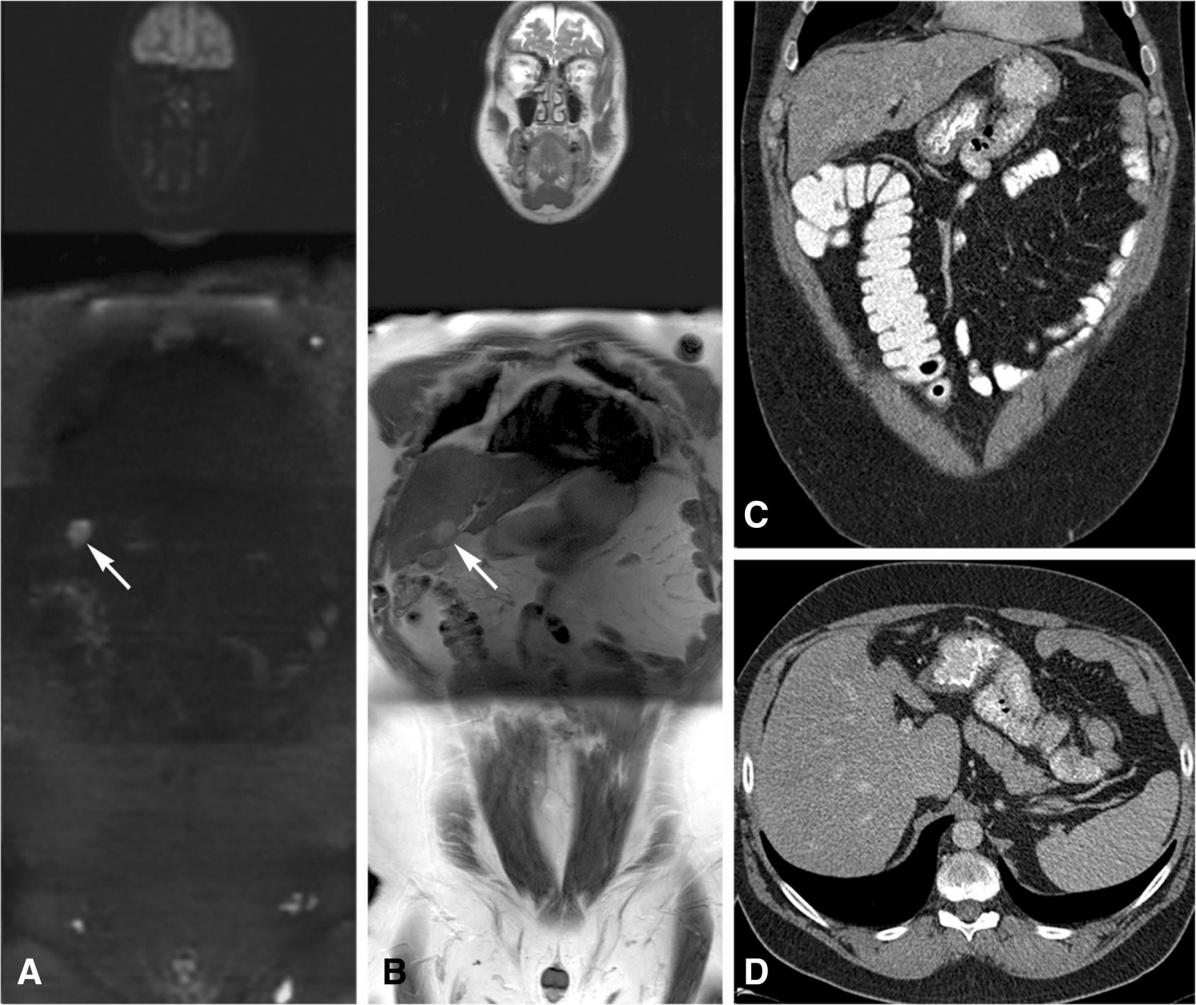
A 49 year old male patient with a history of a sigmoid resection because of adenocarcinoma was diagnosed with tumour recurrence. He had a WB-DWI/MRI for operability assessment. Apart from limited peritoneal disease (not shown on the images) a liver metastasis was found in liver segment 4B, shown with the arrows on the coronal b1000 DWI (a) and the coronal T2-weighted image (b). This liver metastasis was not recognised on the axial (c) and coronal (d) CT images. The patient could undergo a curative debulking with RFA of the liver metastasis
